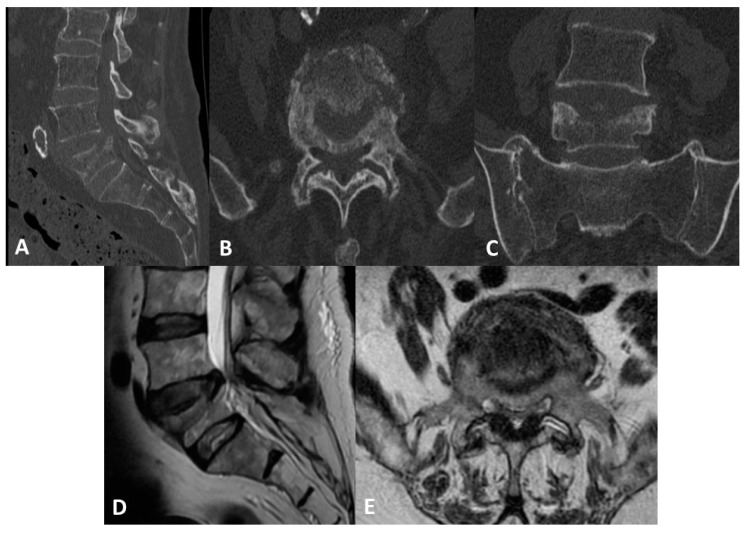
(A) Sagittal CT scan of the L5 fracture; (B) axial CT scan at the fracture level; (C) coronal CT scan; (D) sagittal MRI scan of the L5 fracture; (E) axial MRI scan at the fracture level.
